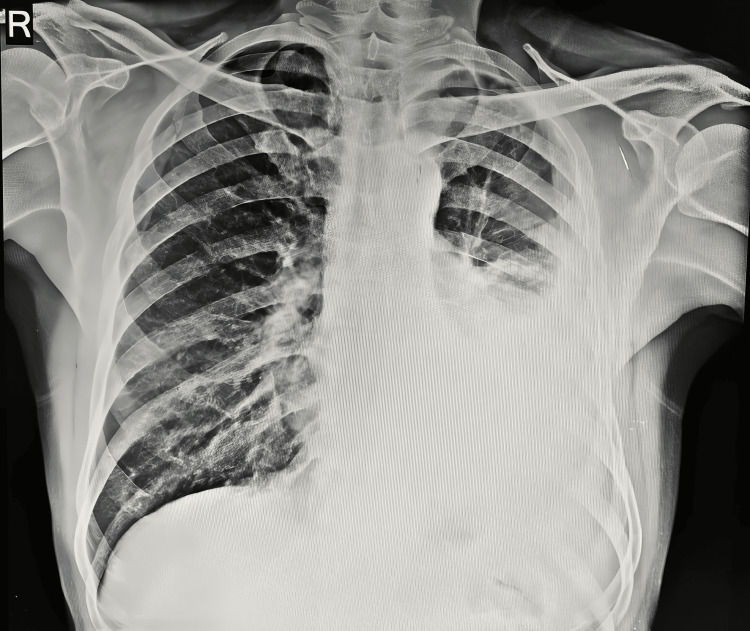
Chest X-ray showing left M2 + L2 homogeneous opacities with blunting of costophrenic angle.
